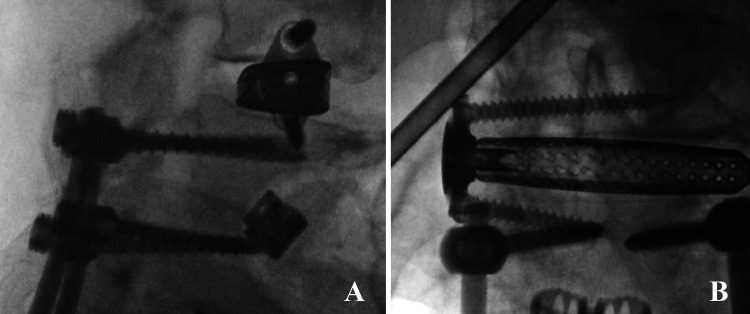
Lateral (A) and Anterior-posterior (B) Lumbar X-ray Demonstrating Placement of the L3-4 Interbody Spacer. Postoperative lateral lumbar x-ray demonstrating final placement of L3-4 interbody spacer.
